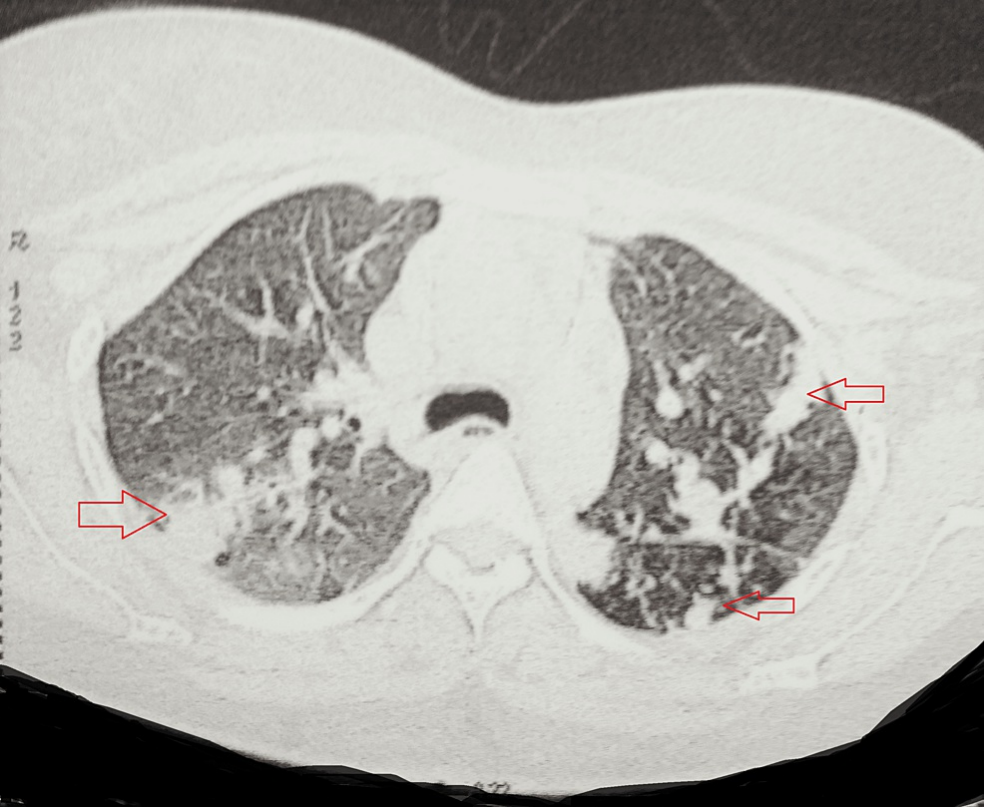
HRCT of the chest showing multiple bilateral patchy consolidations HRCT: high-resolution computed tomography
